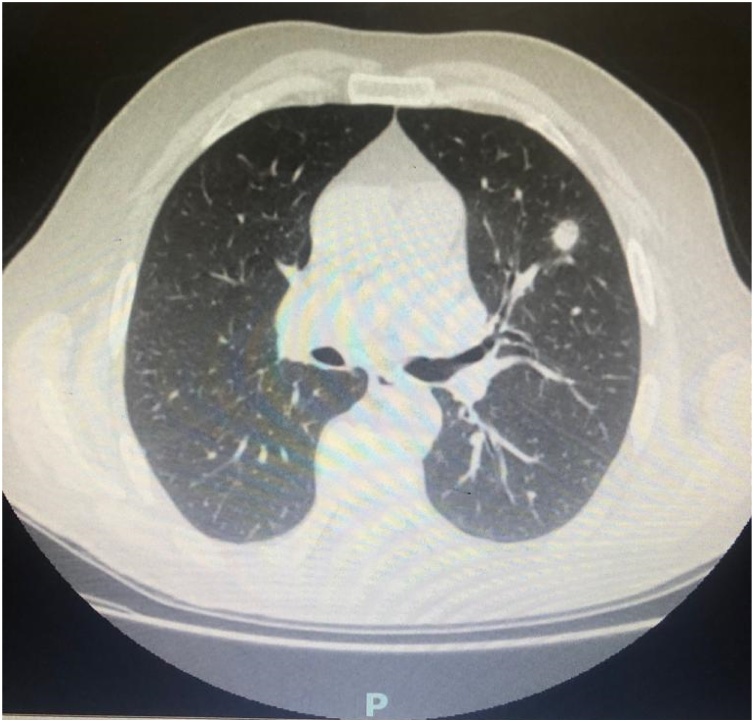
CT imagine of pulmonary metastasis.
